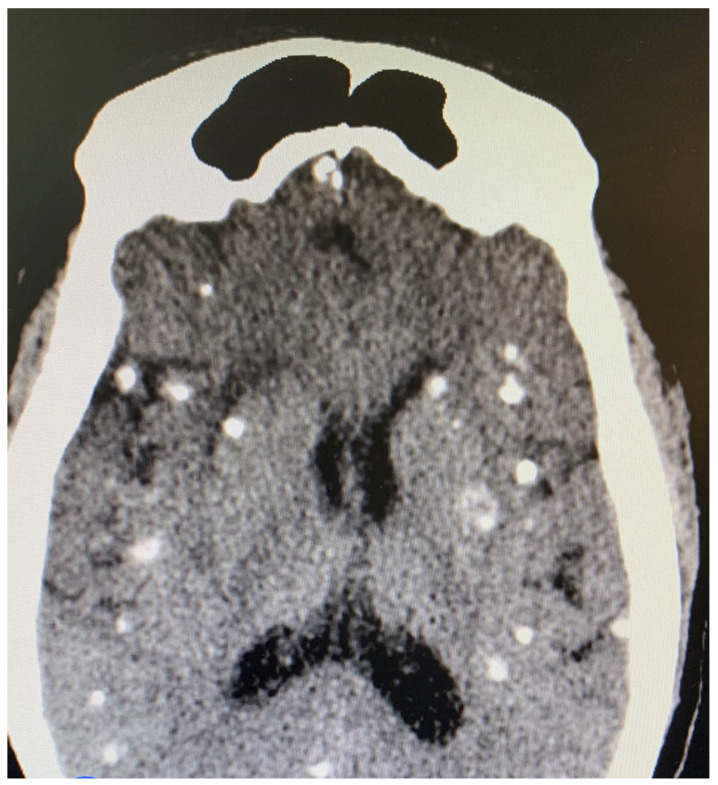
Head CT scan showing multiple cysticerci in the cerebral cortex.
